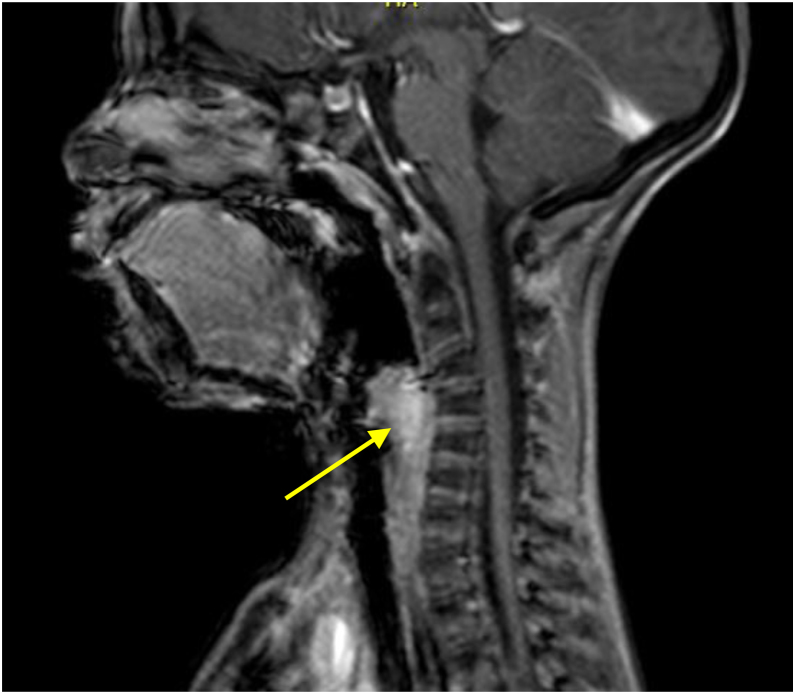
Cervical MRI, in sagittal plane. Arrow point to a well-defined nodular supraglottic expansive lesion, centered at the left aryepiglottic fold, presenting enhancement after contrast, without restriction to diffusion, measuring 2.5 × 1.8 × 2.4 cm.
